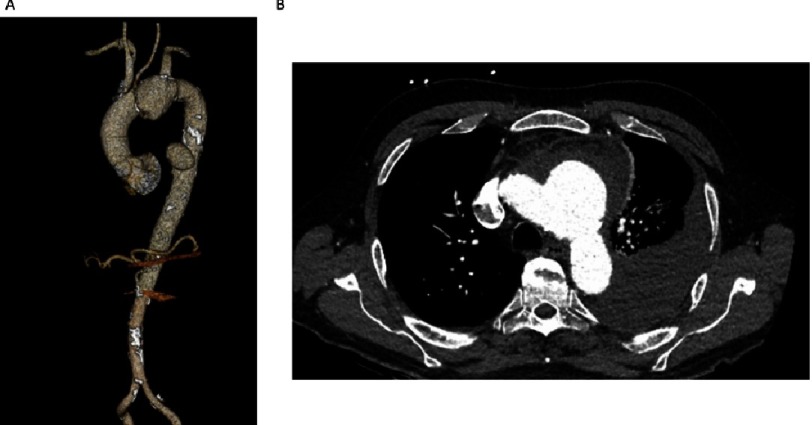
Pre-operative CT angiography of the thoracic aorta. (A) Between the origins of the left common carotid and left subclavian arteries (LSCA), there was a 3.7 × 4.4 × 5.2 cm aneurysm directed towards the left, inferiorly and posteriorly. A second 4.3 cm saccular aneurysm arose from left lateral wall of the descending thoracic aorta. (B) The presence of peri-aneurysmal fluid, left hemothorax, and hemopericardium as well as his clinical presentation, confirmed relatively acute contained rupture (Figure 1B).
